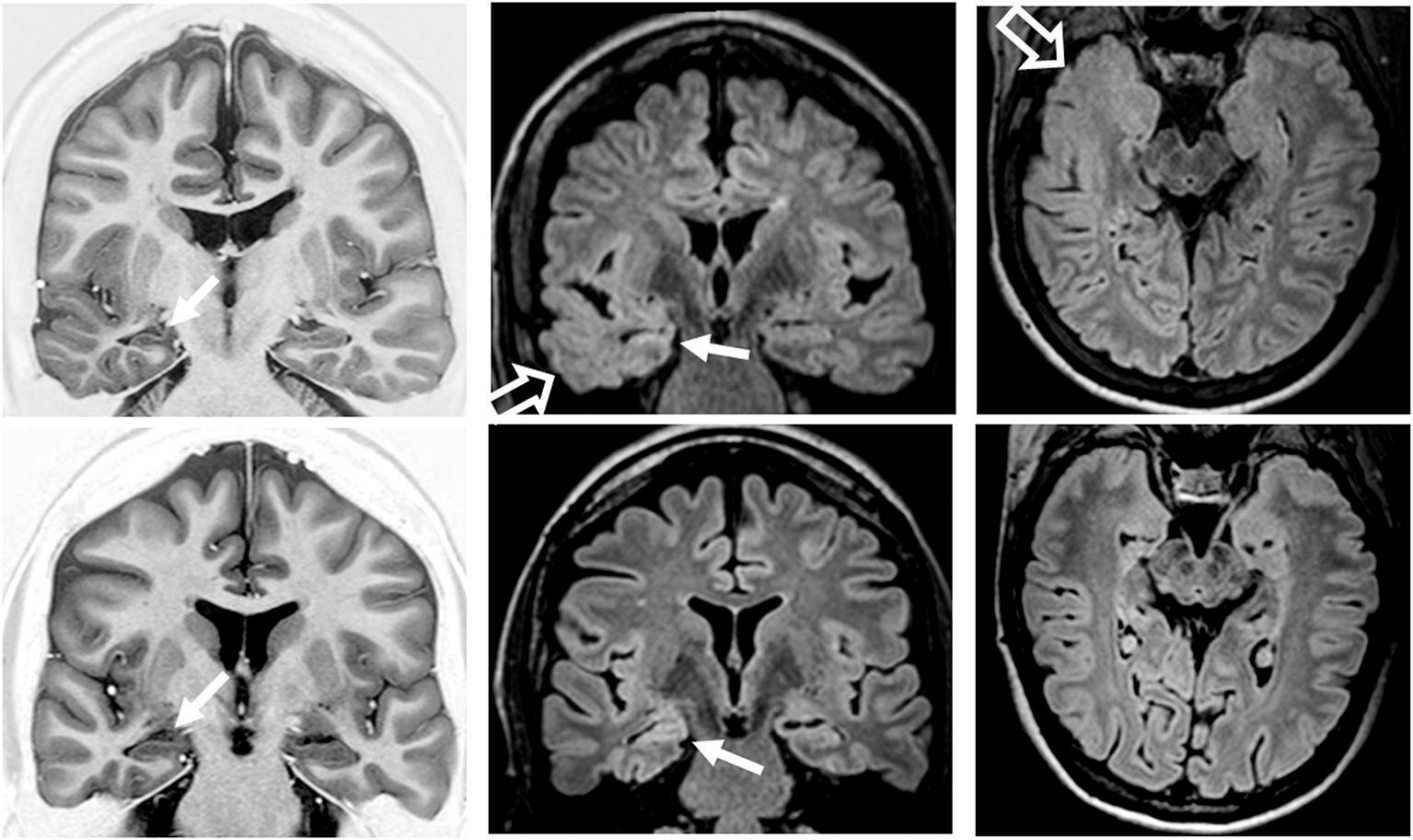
Preoperatory coronal T1-weighted inversion recovery and coronal and axial fluid-attenuated inversion recovery (FLAIR) images from patient 2 (top row) with temporopolar blurring in FLAIR images (open arrows) and from patient 6 (bottom row) without temporopolar blurring. Both patients had MRI signs of right hippocampal sclerosis (atrophy and hyperintense FLAIR signal; arrows).
